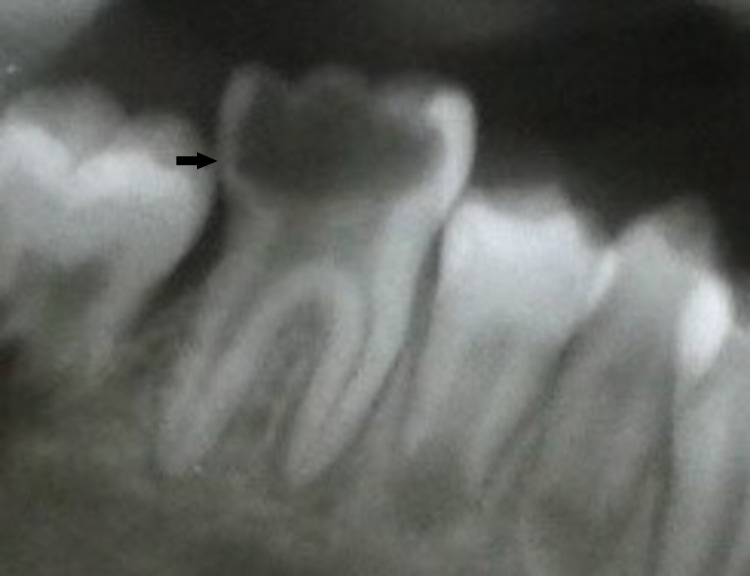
Preoperative X-ray 46: coronal radiolucency extending to the pulp chamber and periapical radiolucency present in mesial and distal roots
